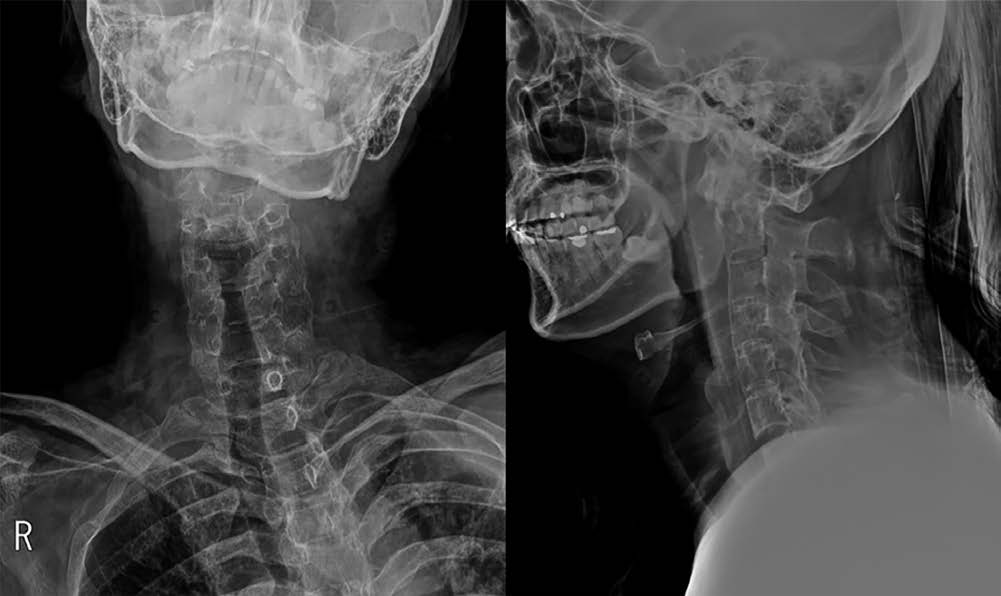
Preoperative X-ray showing that patient’s head was rotated to the right and there was an anterior subluxation of the C1 and odontoid tip relative to C2.
